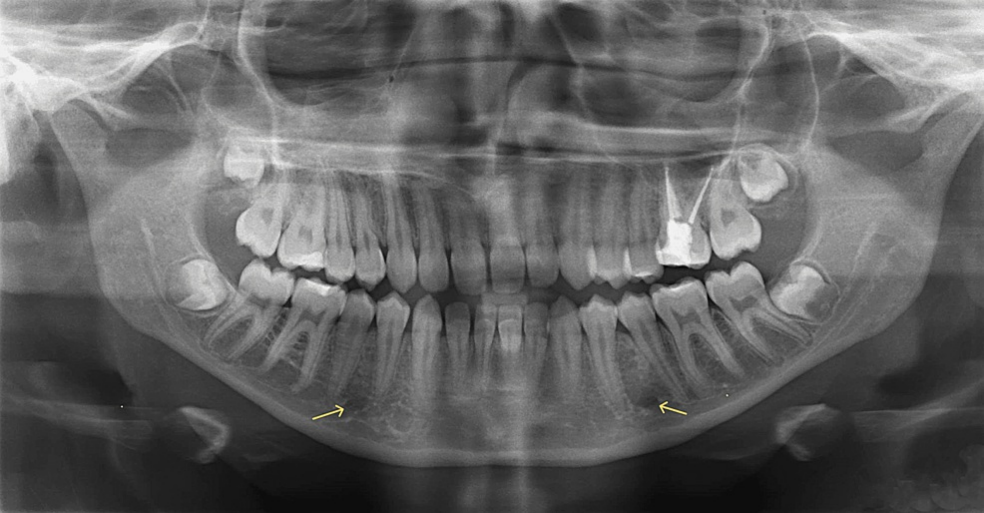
Panoramic radiograph showing MF on the third position and shape (yellow arrows) MF: mental foramen
